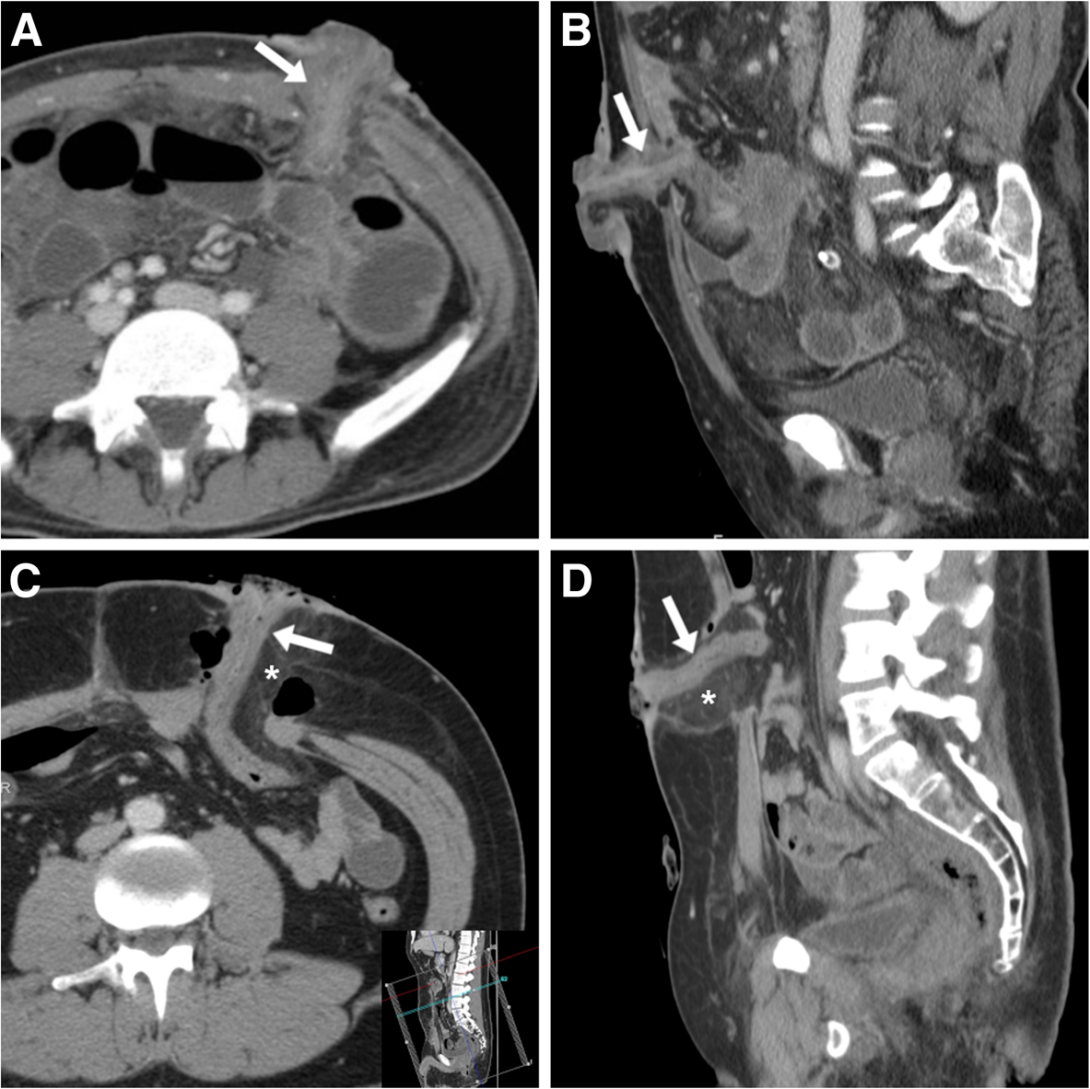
Expected early CT findings in newly created stomas. a, b A typical recent ileostomy, with collapsed stomal tract (arrows) showing mild uniform mural stratification (enhancing mucosa and oedematous submucosa). c, d Uncomplicated descending colostomy created 4 days earlier during low anterior rectal resection, with stomal tract (arrows) showing similar features to a, b, surrounded by oedematous fat stranding (*) and gas bubbles as expected postsurgical findings
